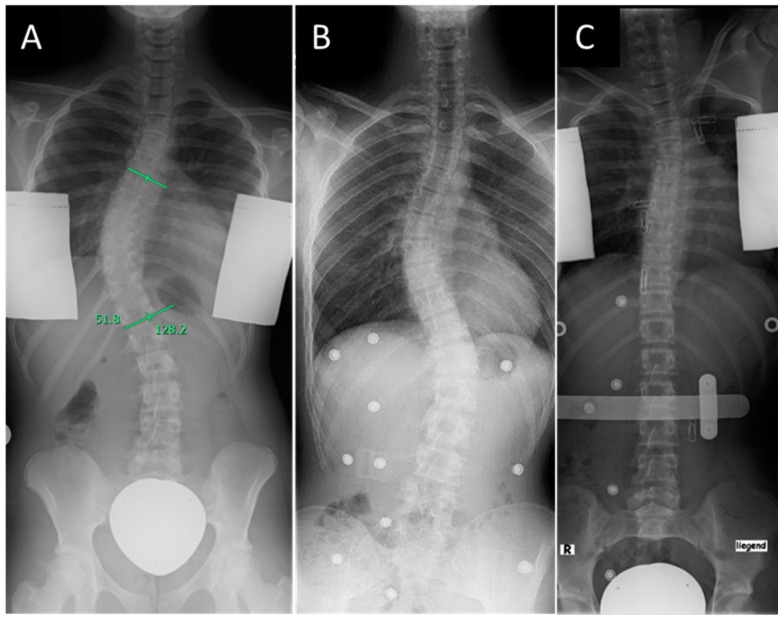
Right thoracic idiopathic scoliosis of Lenke type 1 identified in a standing radiograph (A), standing radiograph to assess the correction of the curve in the Chêneau corset (B), and supine radiograph to assess the correction of the curve in the Charleston Bending Brace (C).
